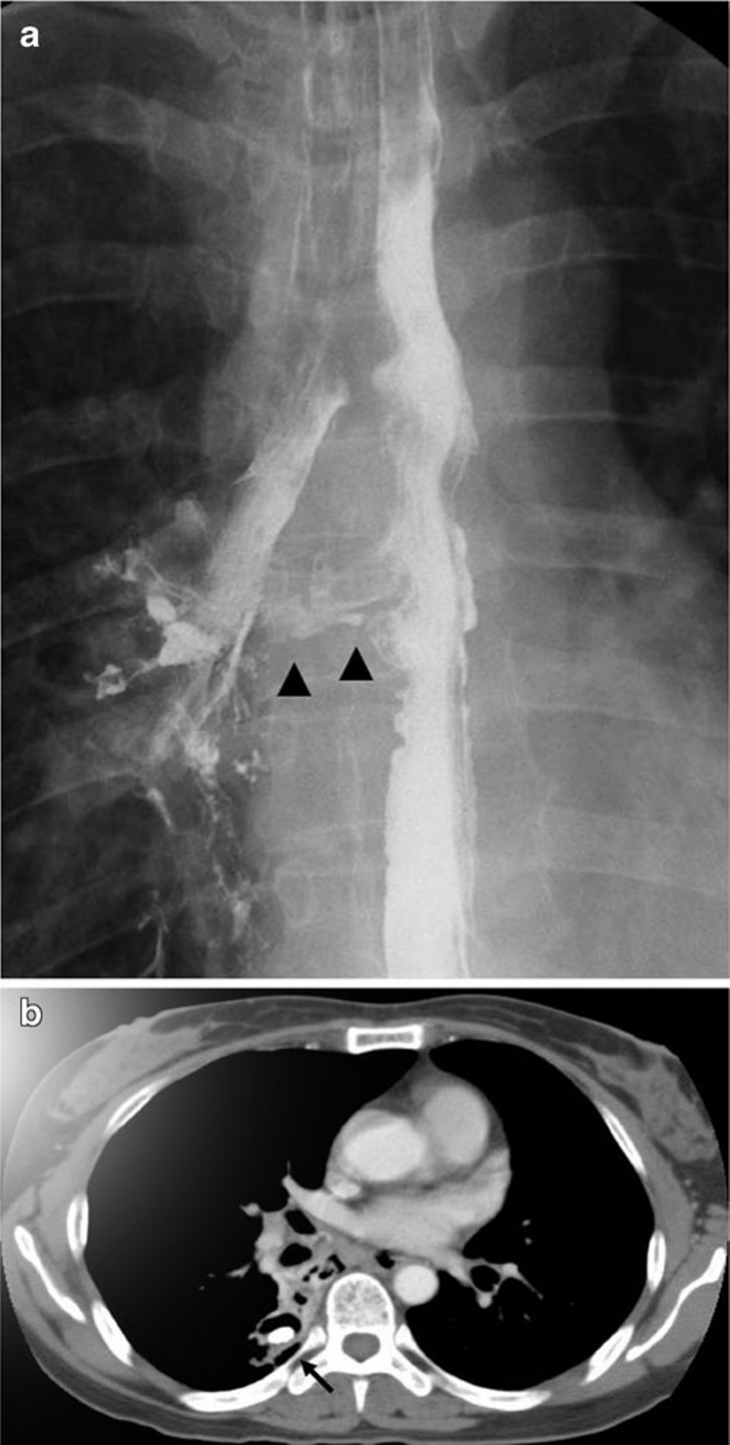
Actinomycosis with oesophagobronchial fistula in a 44-year-old woman who complained of a repetitive cough with food intake. a Barium oesophagography depicts the fistula tract (*arrowheads*) between the oesophagus and right bronchus intermedius. b Chest CT scan shows a segmental consolidation and bronchiectasis containing a broncholith (*arrow*) in the right lower lobe of the superior segment. Consolidation extends to the mediastinum and oesophagus
